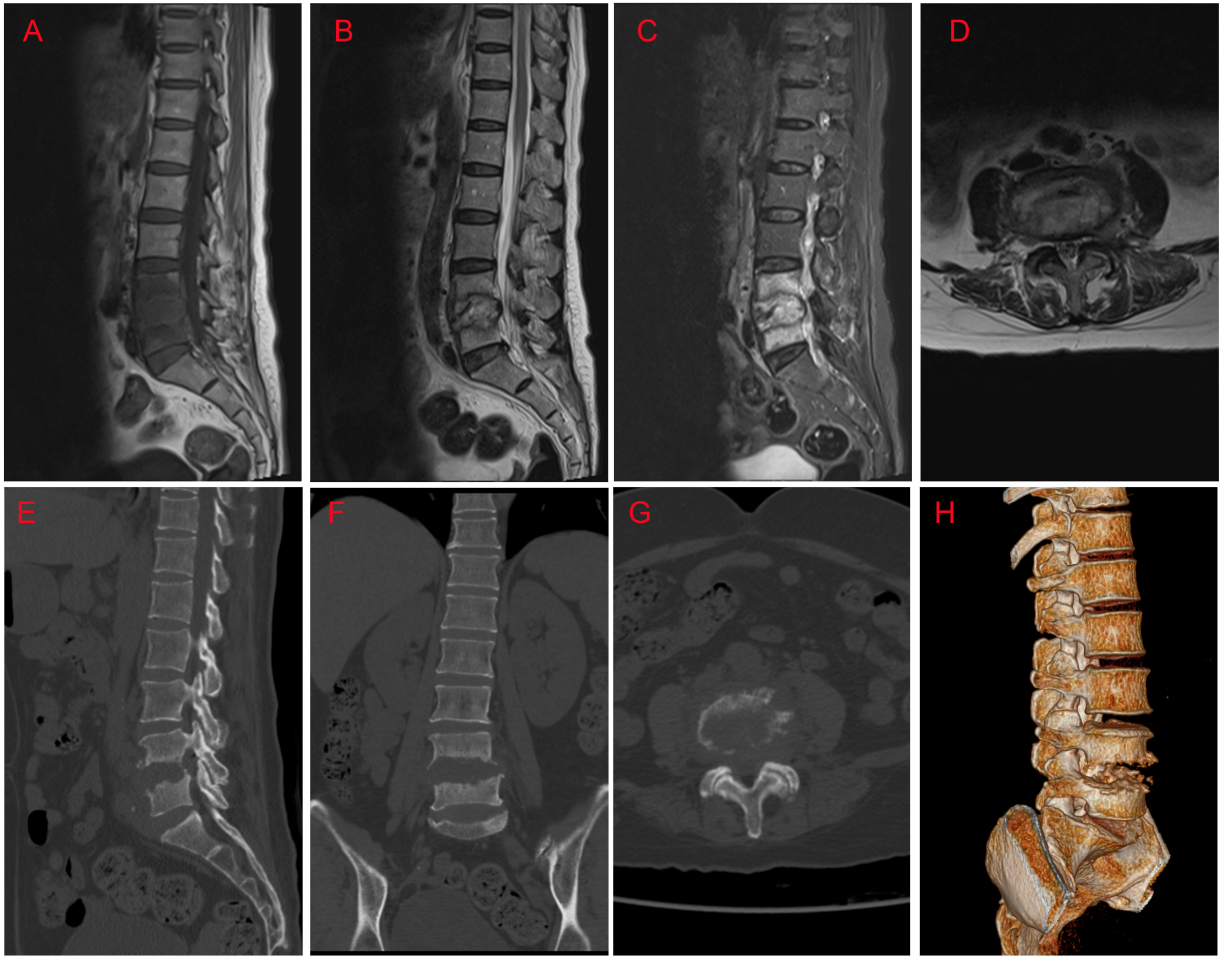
MRI and CT findings at approximately 24 days after antifungal therapy. (A) Sagittal T1-weighted image; (B) Sagittal T2-weighted image; (C) Sagittal T2-weighted fat-suppression image; (D) Axial T2-weighted image at the level of L4/5; (E) Sagittal CT image; (F) Coronal CT image; (G) Axial CT image; (H) Preoperative CT three-dimensional reconstruction image.
